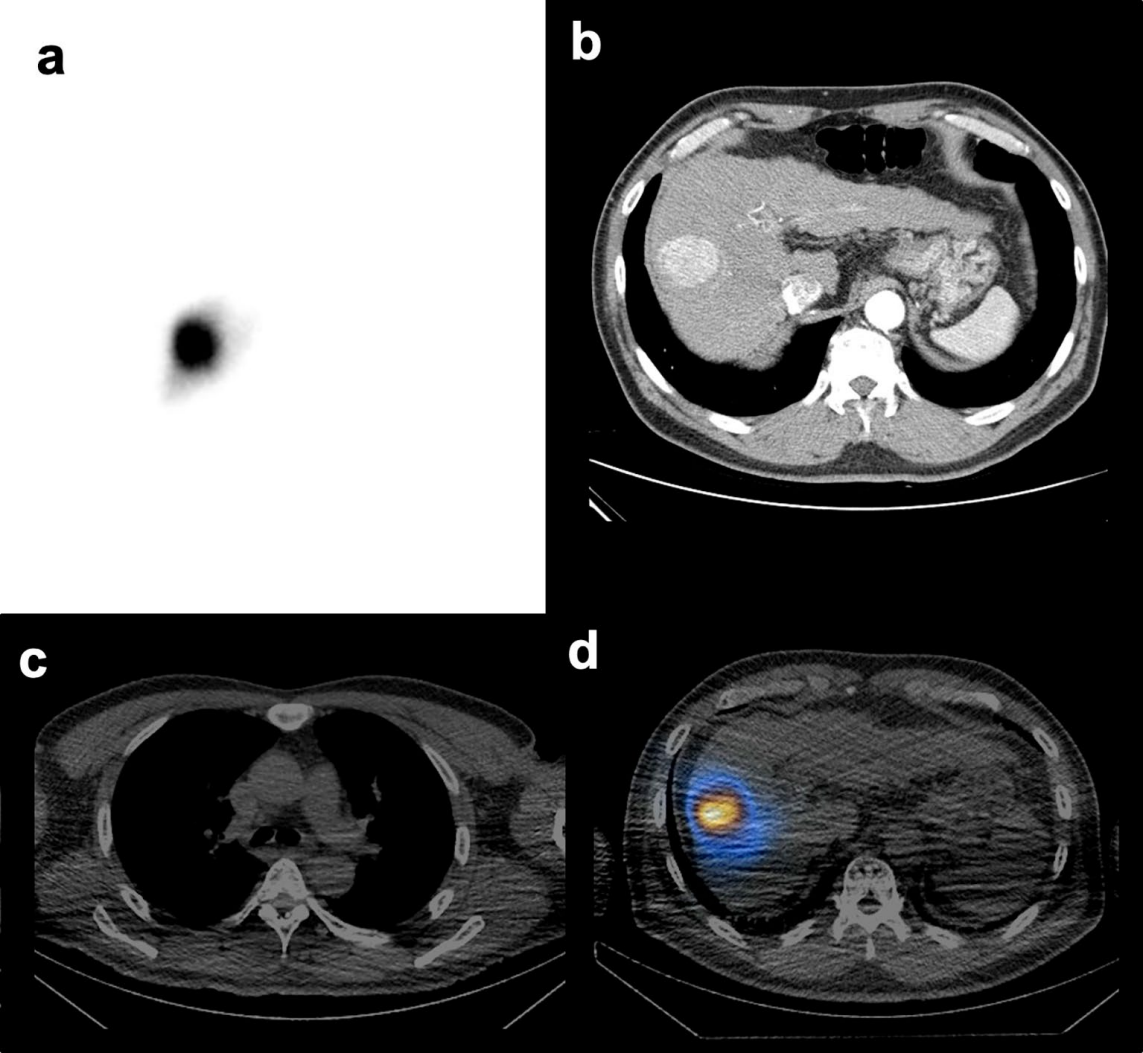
Representative cases of a patient with low LSF. The patient (58-year-old male) had an LSF of 2.7% on the planar scan (a), with a smaller sized tumor (36 ml), with an integral total (HU × L) value of 4.08 (b). From the SPECT/CT, LSF was 3.2% (c), and the tumor uptake was 87.5% (d).
